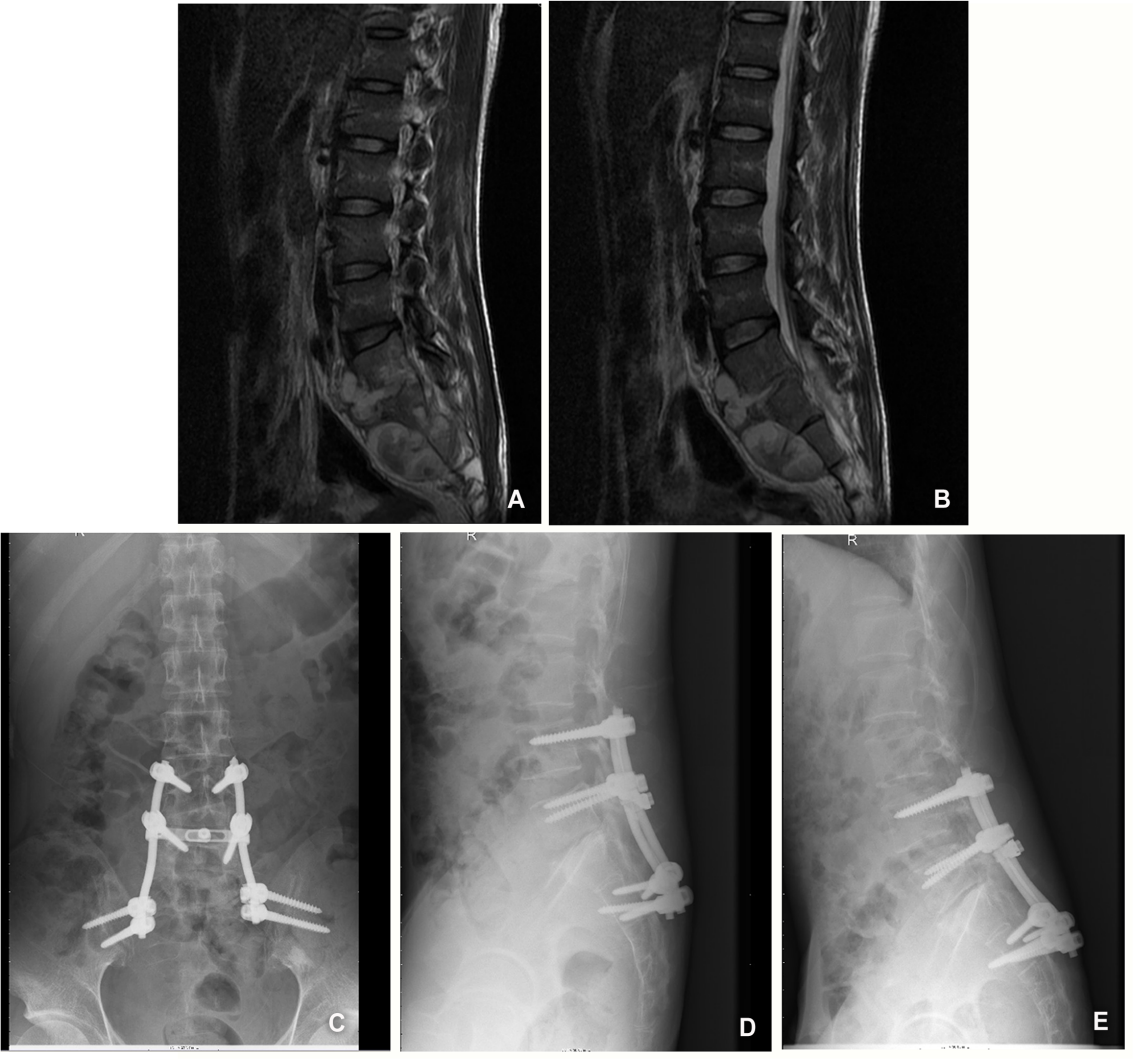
A 28-year-old man with lumbosacral spinal tuberculosis underwent anterior debridement, decompression combined with posterior plate fixation. (A,B)Pretreatment MRI shown destruction of the L4–S1 vertebrae and paravertebral abscess with concomitant compression of the spinal cord.(C) Immediate postoperative radiographs demonstrating anterolateral debridement, bone graft and internal fixation. (D,E) At 22 months’ follow-up, plain X-ray showed maintenance of the correction and solid fusion.
